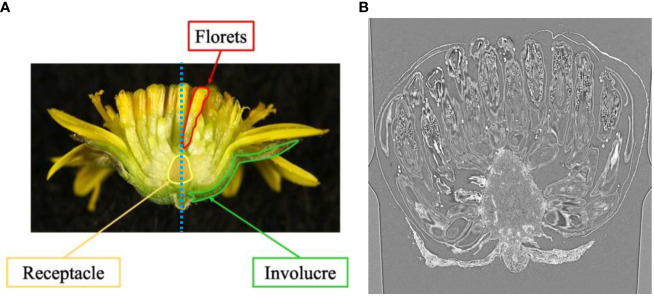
Example of mid-longitudinal section (A) and CT slice image of *C. seticuspe*
